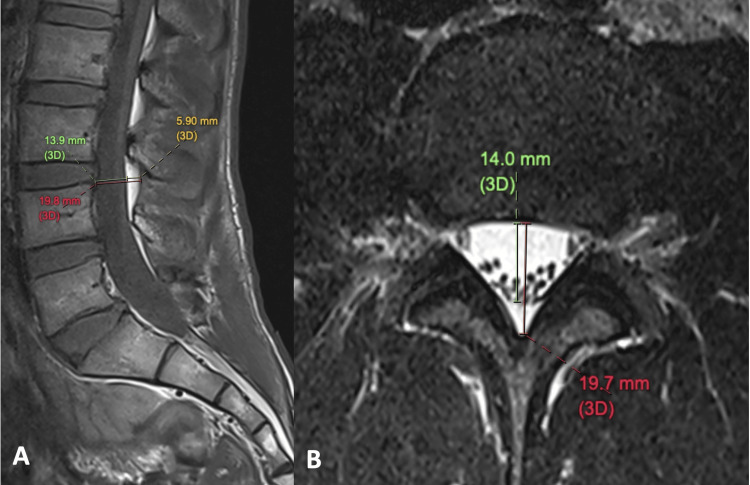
(A) Sagittal T1-weighted and (B) axial T2-weighted MRI of lumbosacral spine on a 30-year-old man with chronic low back pain. Red line: AP length of spinal canal; green line: AP length of dural sac; yellow line: AP length of epidural fat. MRI: magnetic resonance imaging.
